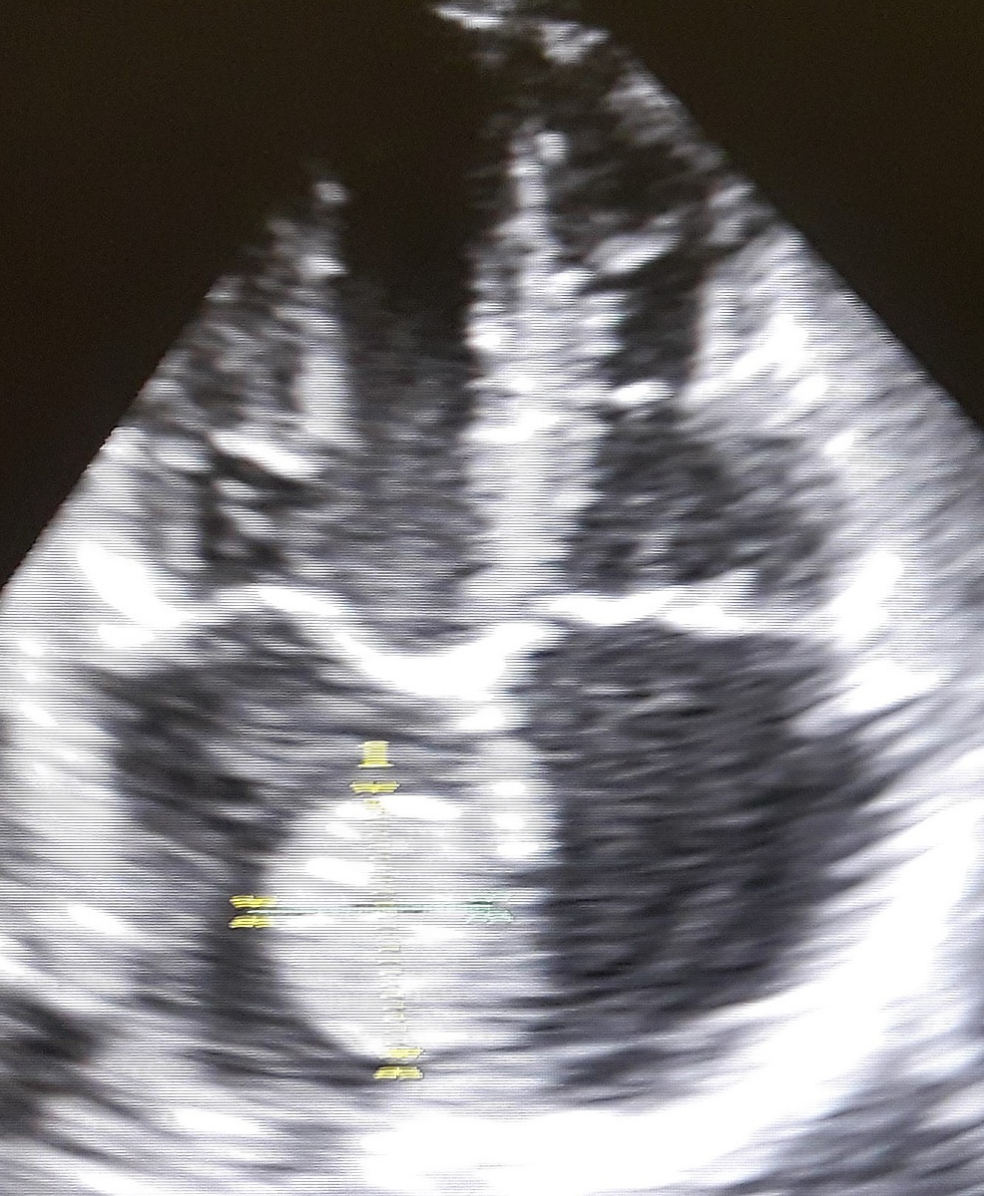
Myxoma in the left atrium. Transthoracic echocardiographic study, apical 4 chamber view. Left atrial mobile homogeneous hyperechoic mass, attached to interatrial septum.
